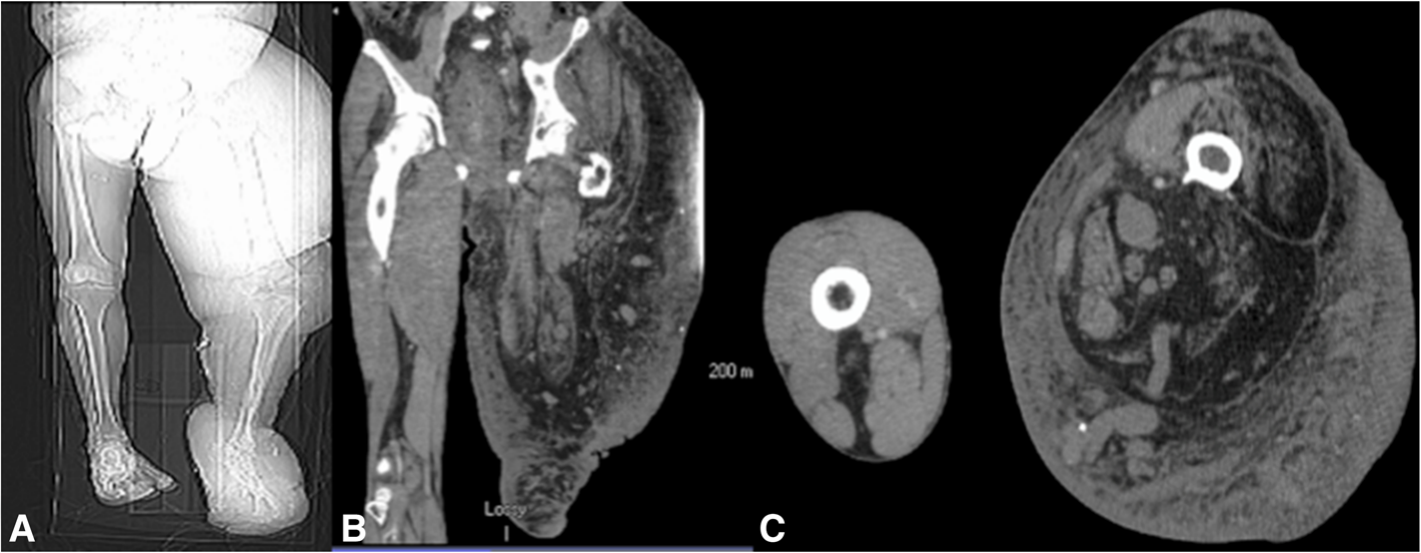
Pilot image (A) and the computed tomography scan with coronal (B) and axial (C) cuts of the left lower extremity showing hypertrophy of the left leg.
